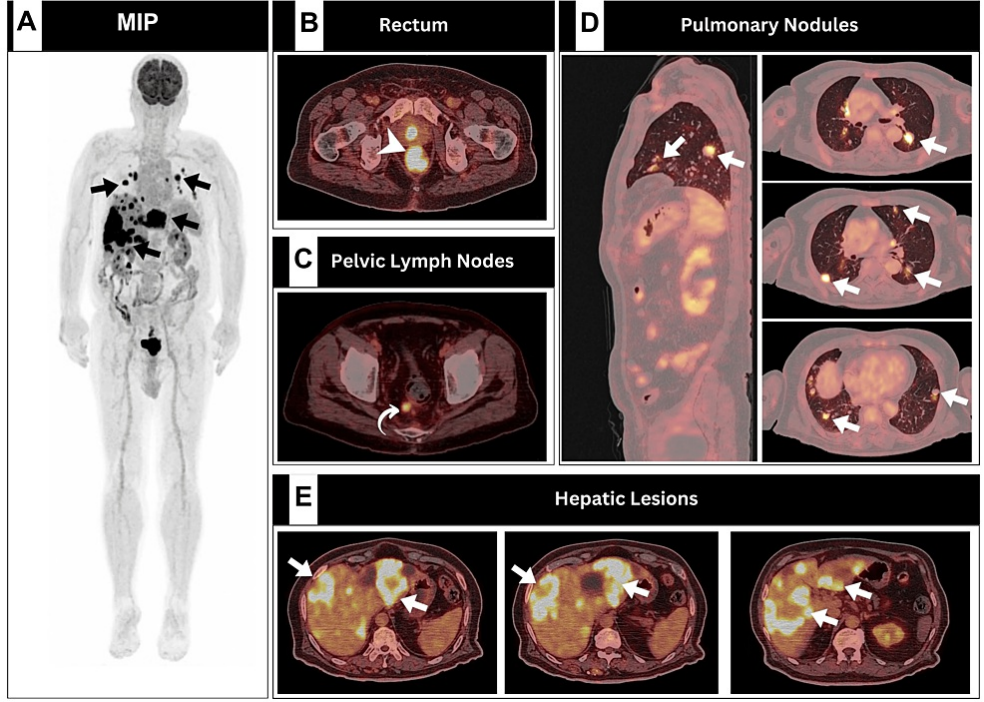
Baseline [18F]FDG PET/CT performed for the detection of unknown primary and subsequent staging A: Maximum intensity projection. B: Rectal transaxial PET/CT. C: Pelvic transaxial PET/CT. D: Sagittal and transaxial thoracic PET/CT. E: Hepatic transaxial PET/CT images revealed evidence of hypermetabolic primary rectal malignancy (arrowhead) associated with a few hypermetabolic perirectal lymph nodes (curved arrow) and multiple metastatic hepatic and pulmonary lesions (arrows). [^18^F]FDG PET/CT - [^18^F] fluorodeoxyglucose positron emission tomography/computed tomography
